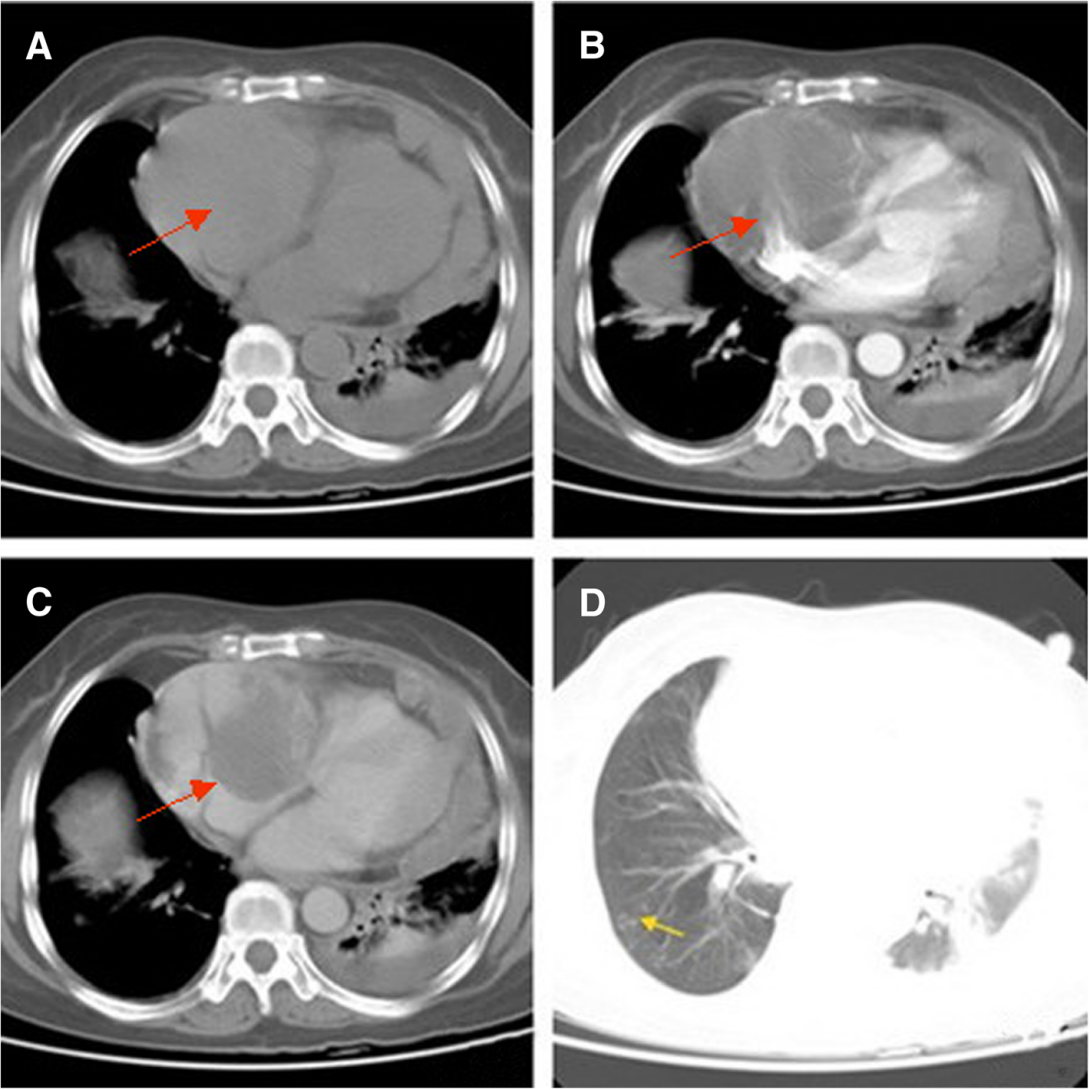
Female, 53 years old, right atrium angiosarcoma (red arrows). a: Plain CT scan shows an equal-density mass in the right atrium. The density is partly homogenous, and pericardial and pleural effusion are noted. b, c: Enhanced CT scan indicates inhomogeneous and centripetal mass enhancement, and multiple nodules are seen on the pericardium. d: Metastatic nodules (yellow arrows) are seen in the right lung
